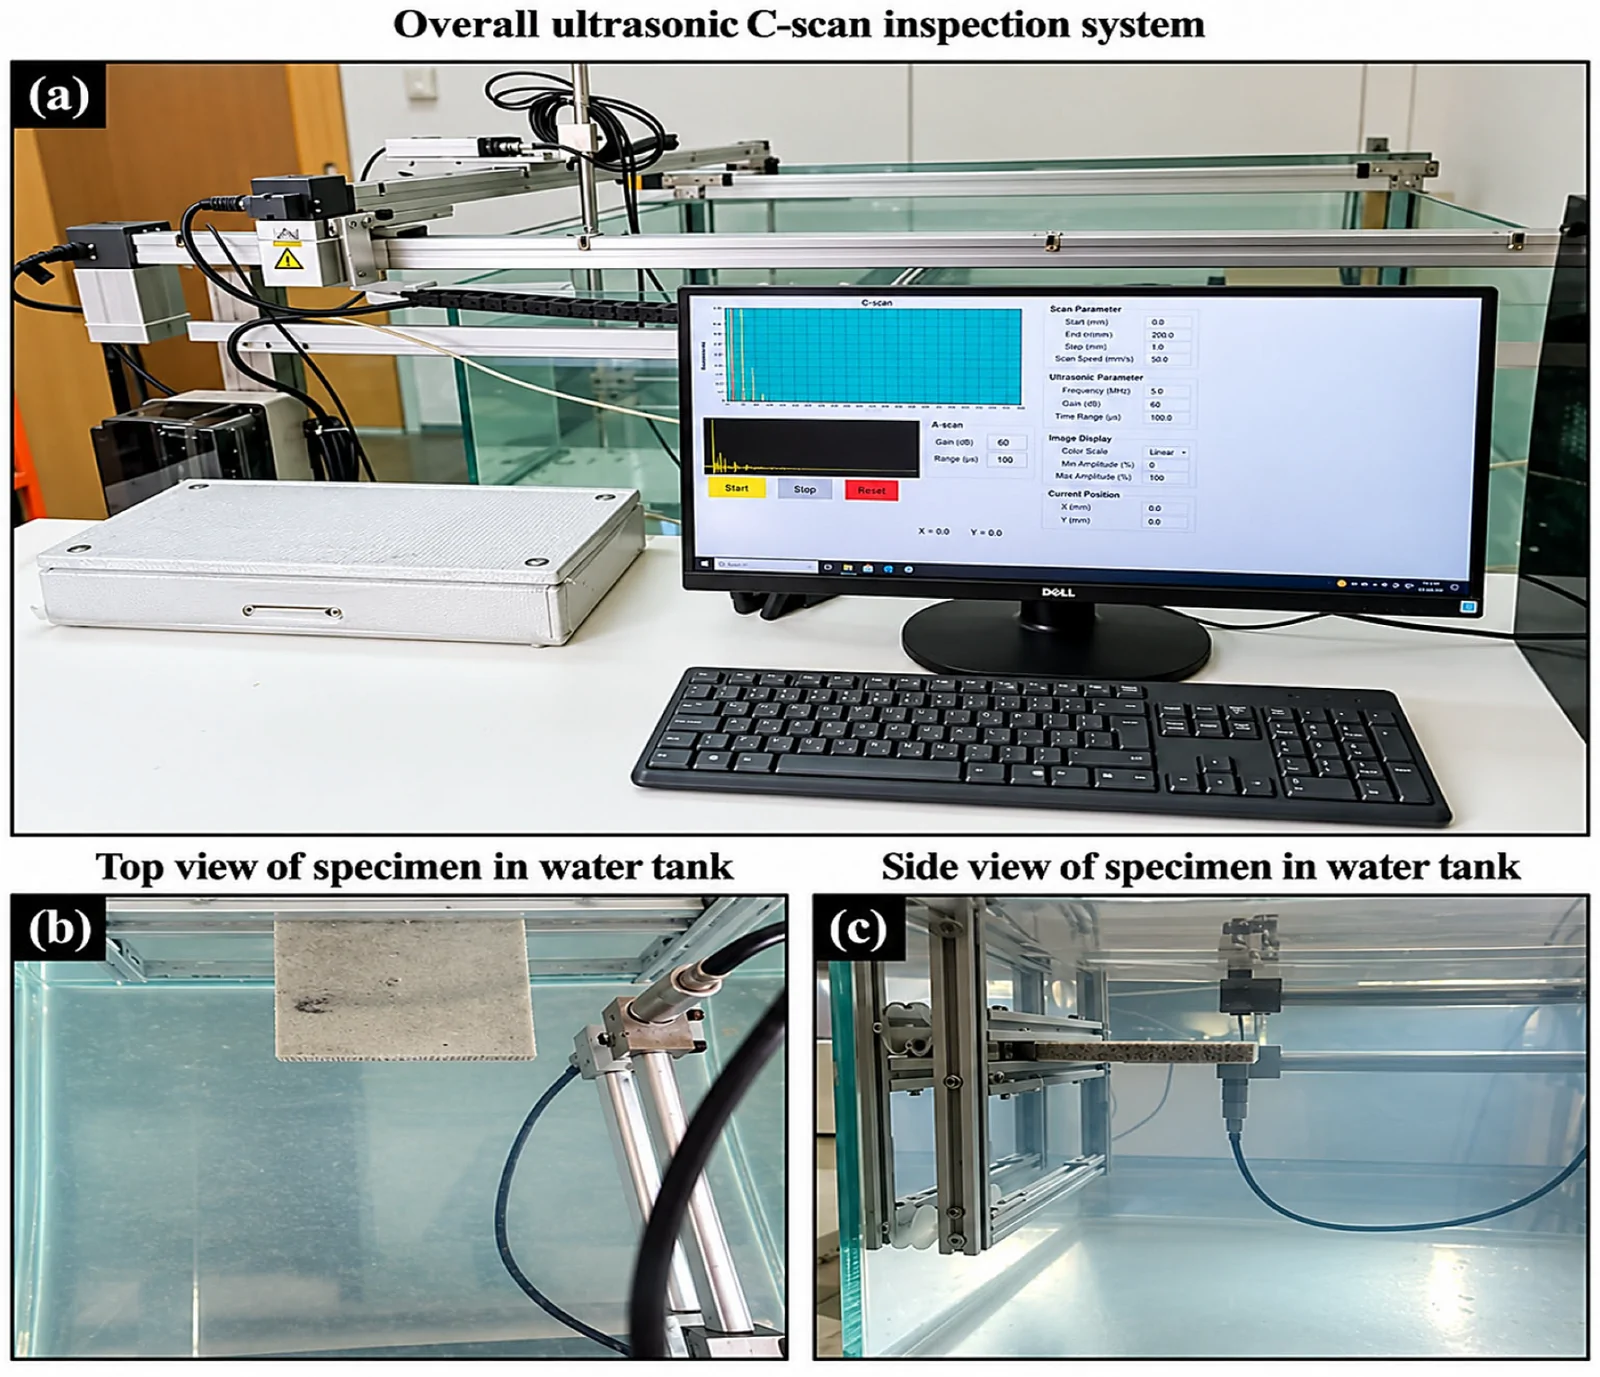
Ultrasonic C-scan analysis system: (a) general view, (b) top view of the sample in the water tank, and (c) side view.
